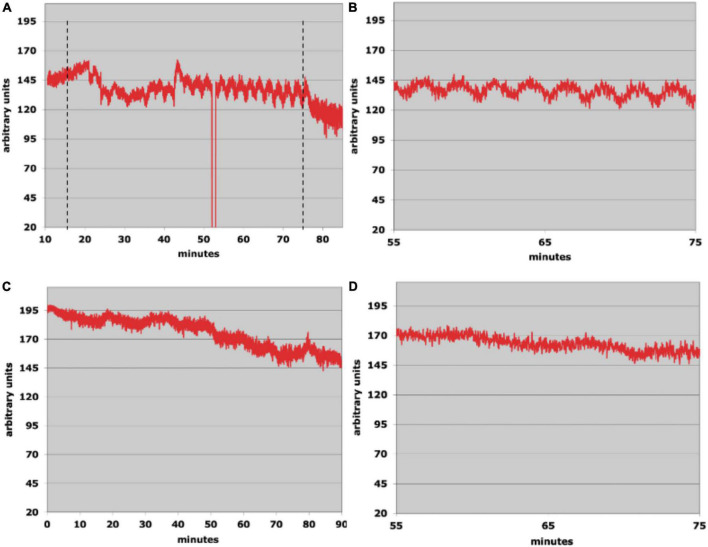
(A) Laser Doppler flowmetry data; the dotted lines show the start and stop times of the time-varying caloric vestibular stimulation (tvCVS) stimulation (the glitch at ∼53 min was due to the recording system falling out briefly and did not affect the measurement otherwise). (B) An expanded view of the oscillations from panel (A). (C) A control run (no tvCVS stimulation). (D) An expanded view of a segment of the control run.
